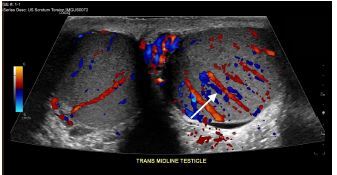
Comprehensive ultrasonographic side-by-side view of testicles after manual detorsion. The image-right corresponds to the patient’s left testicle, which demonstrated hyperemia likely related to detorsion event highlighted by the included arrow. Surrounding edema over affected testicle is also seen.
